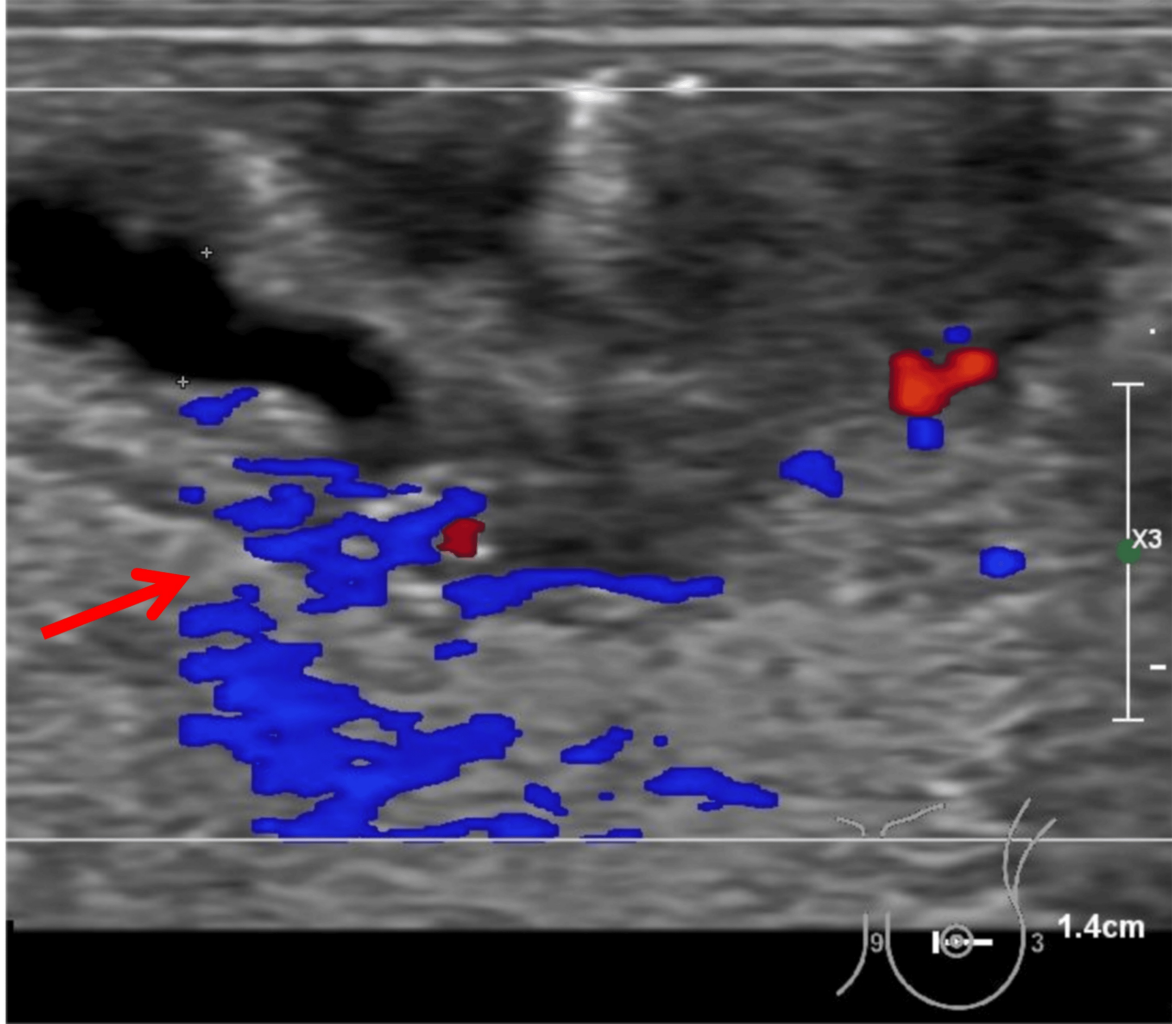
Ultrasonographic Findings of the Left Breast (C) Color Doppler ultrasound image demonstrating a hypoechoic lesion with prominent perilesional vascularity. A dilated duct measuring approximately 2.0mm in diameter is visualized adjacent to the lesion, suggesting a possible ductal connection, which supports the diagnosis of an intraductal papilloma.
